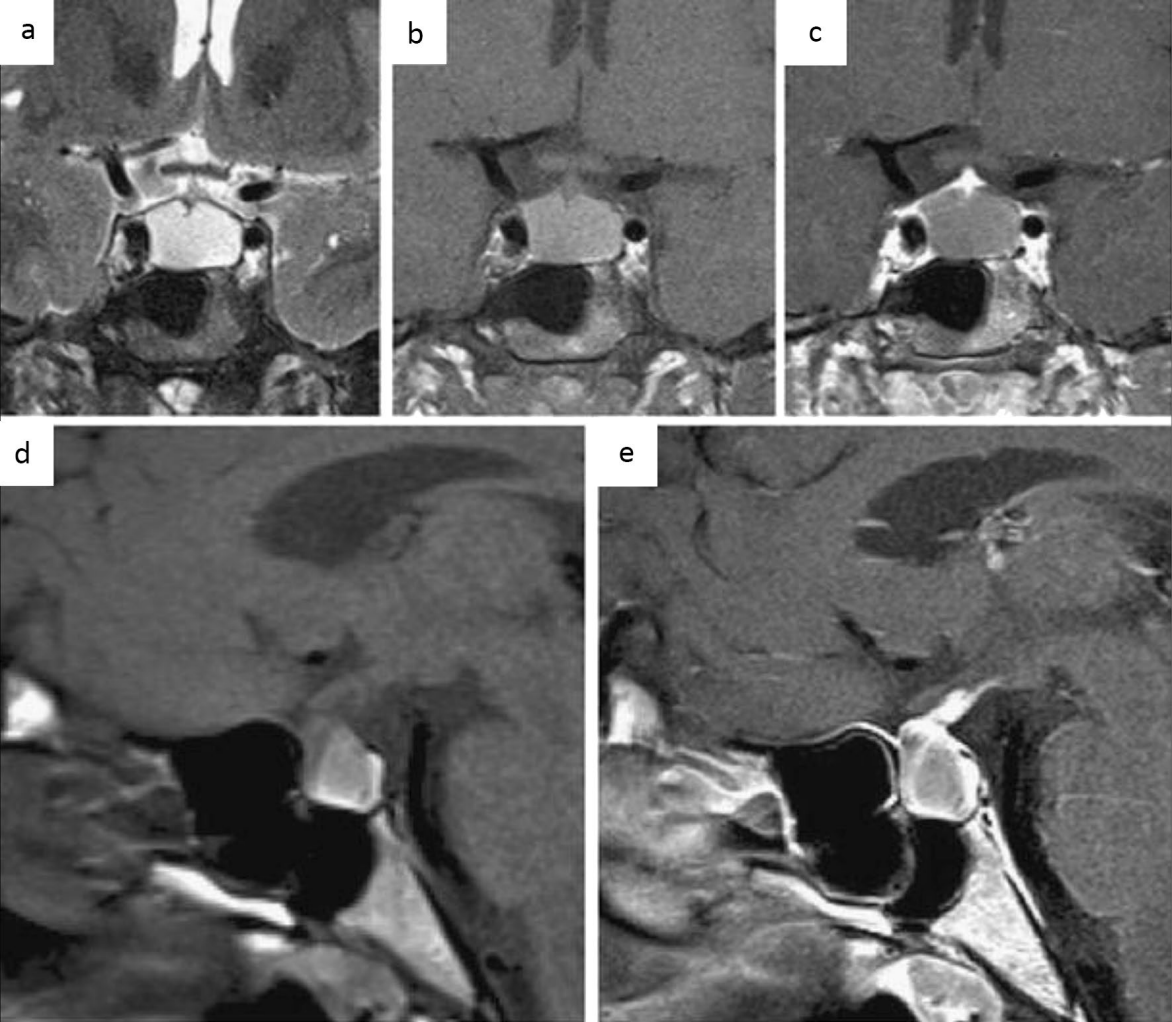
A 20-year-old woman with necrotizing hypophysitis. The enlarged pituitary gland and stalk showing homogeneous hyperintensity on T2- and T1-weighted coronal images a, b with an absence of central contrast enhancement and strong peripheral enhancement c, e are observed. The posterior pituitary T1-weighted bright spot is absent (d). The images were cited from [21]
